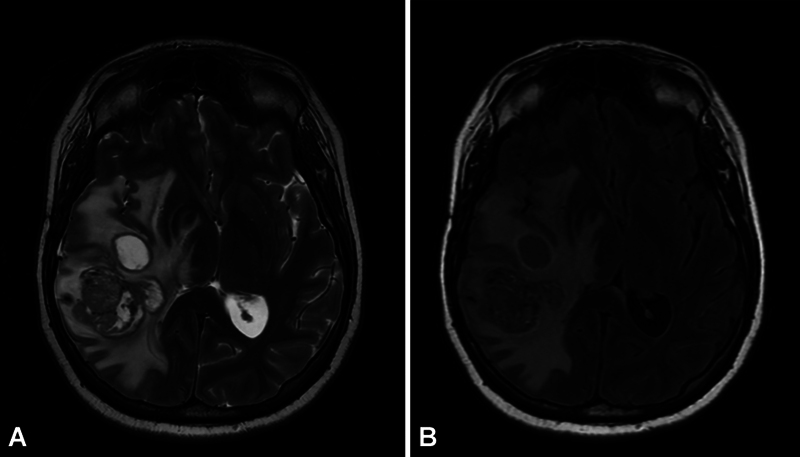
Axial T2-weighted image (
